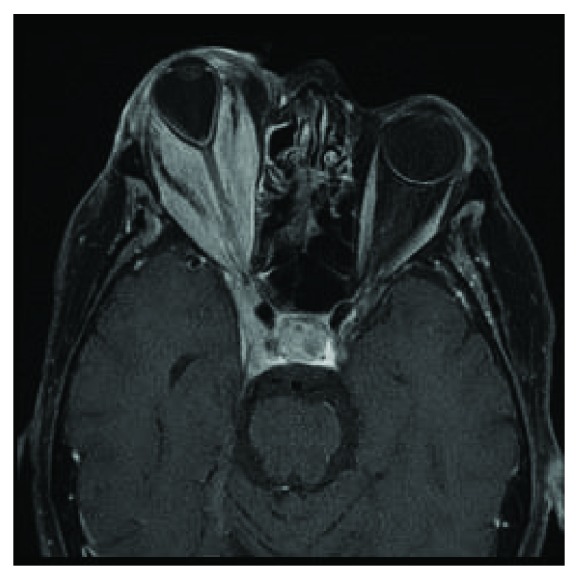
Axial enhanced T1 MRI demonstrating marked proptosis, stretching of the optic nerve, and diffuse enhancement extending into the right cavernous sinus.
